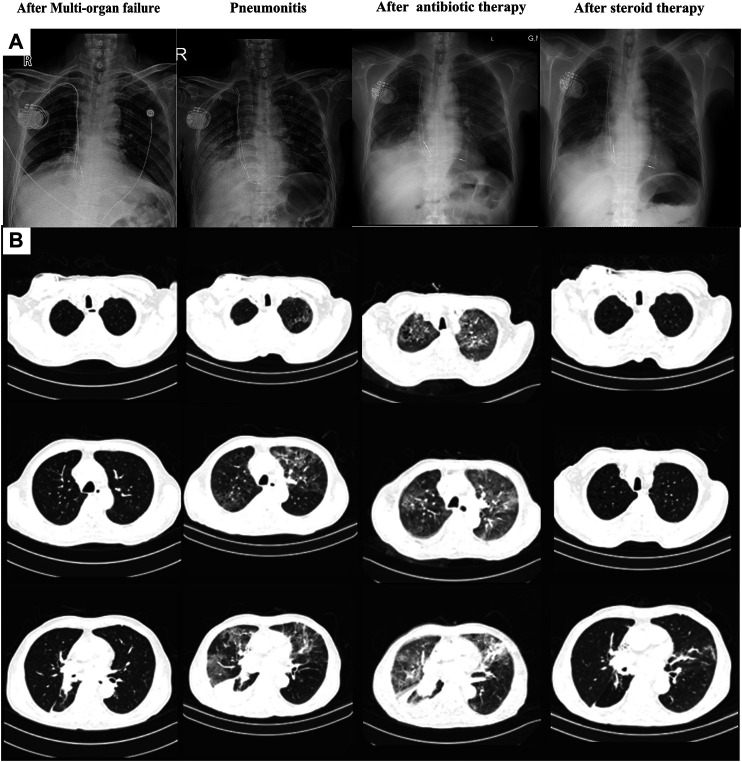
Chest X-ray (A) and computed tomography (B) of the process of pneumonitis finding and treatment.
